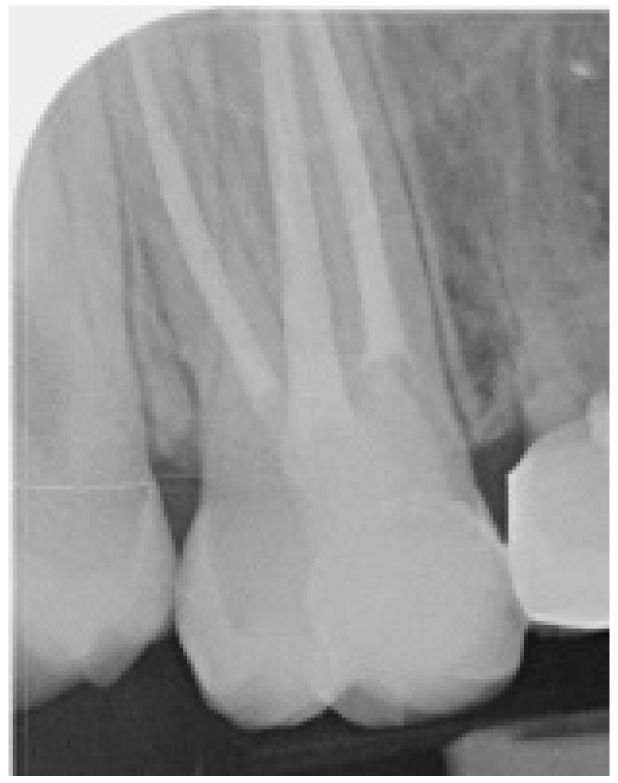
Final X-ray demonstrating marginal integrity and correct fit of the restoration.
